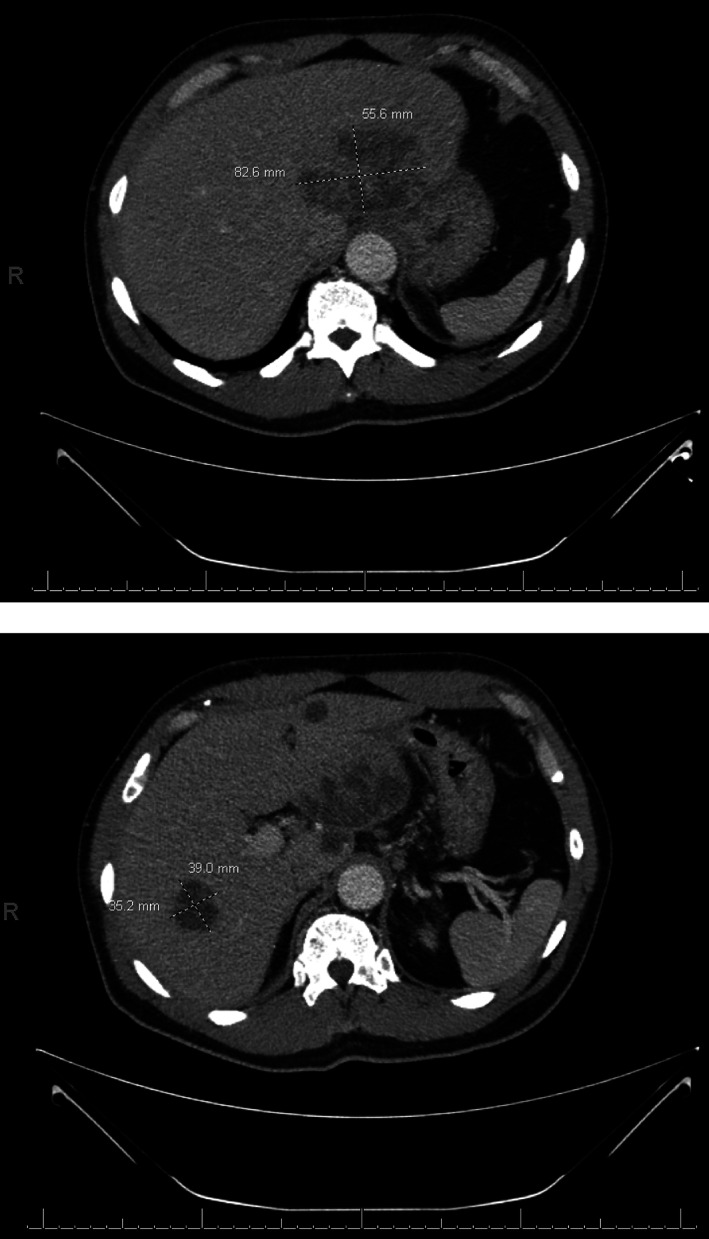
Axial views of contrasted abdominopelvic computed tomography scan demonstrating diffuse, multiloculated hypodense lesions consistent with liver abscesses, with the largest lesions measuring approximately 8.3 × 5.6 cm (top) and 3.9 × 3.5 cm (bottom) in size.
